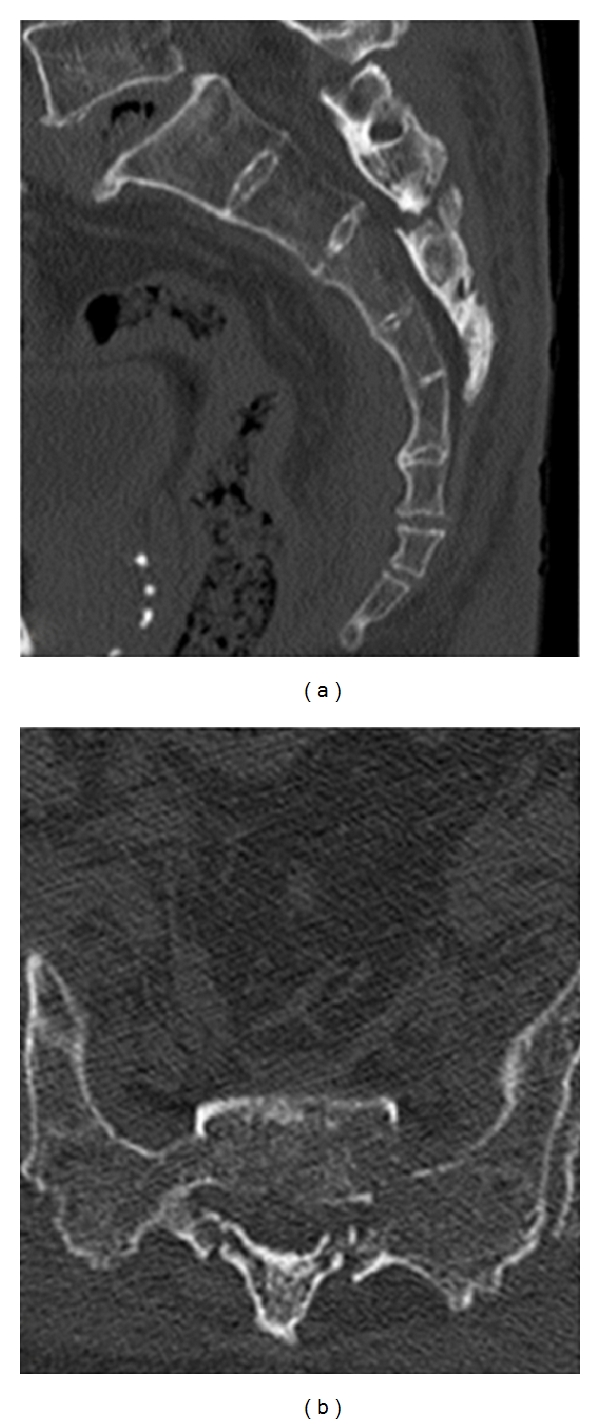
Preoperative reconstructed computed tomogram (CT). (a) Sagittal reconstructed CT: the third sacral lamina fractured and displaced into the spinal canal. The third sacral vertebral body did not angulate. (b) Axial reconstructed CT: the third sacral lamina fractured bilaterally. The spinal canal area decreased. The sacrum was osteoporosis.
